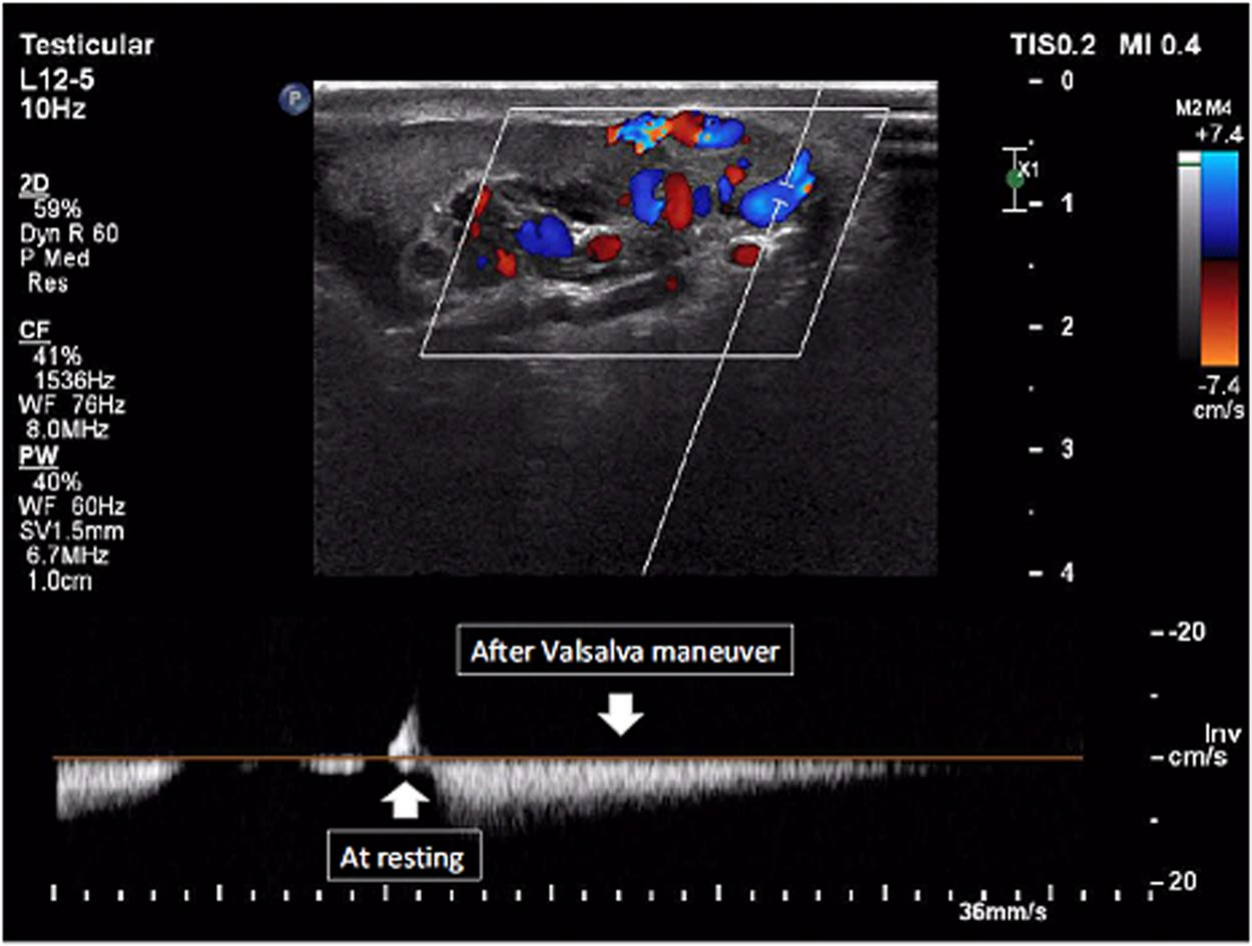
Retrograde pattern reflux: During the Valsalva maneuver, a change in color was seen (from red to blue and vice versa), and in Doppler spectrum, venous flow reversal was seen.
